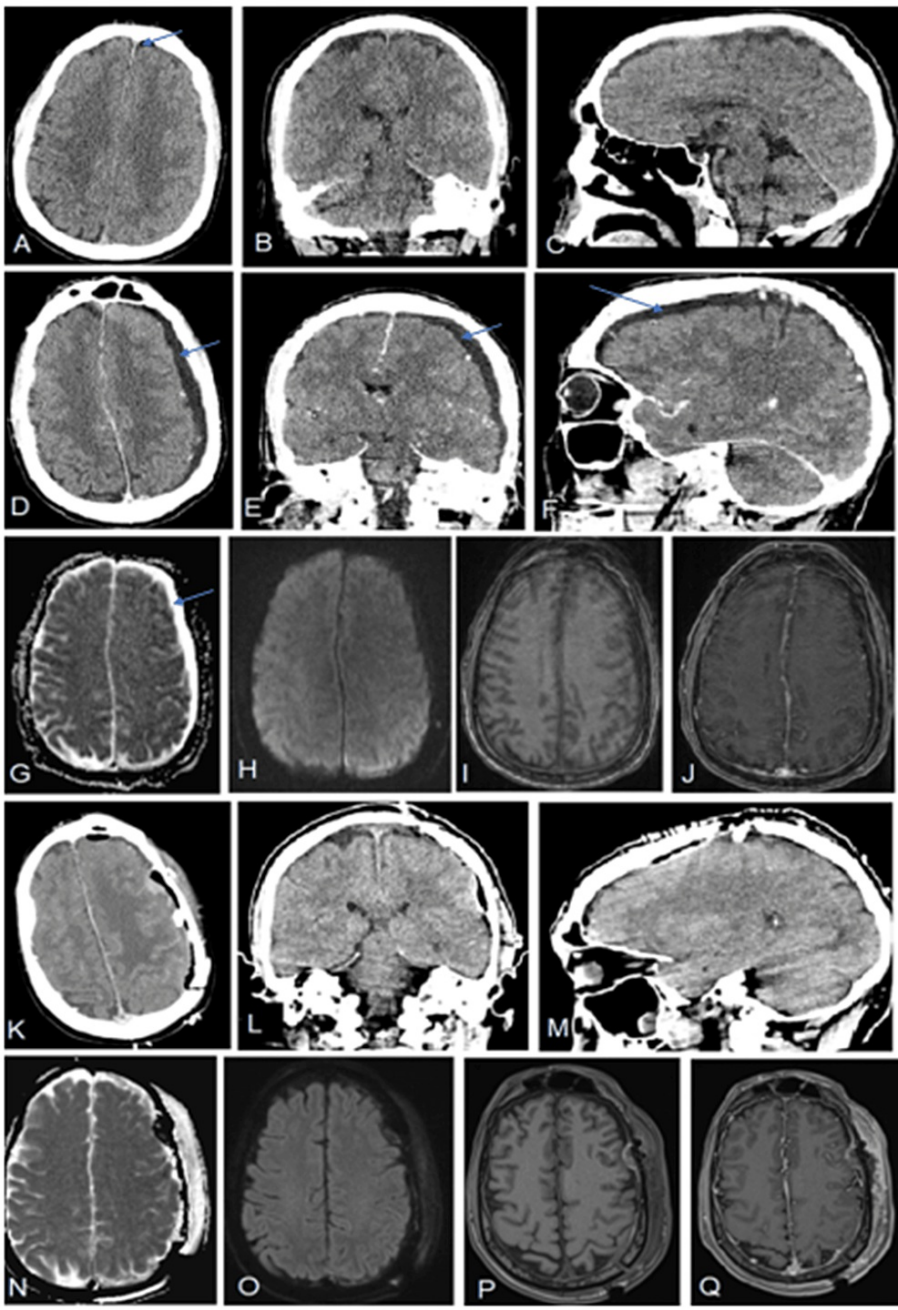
Radiographic images of a non-enhancing subdural empyema in a previously healthy 48-year-old male Figure 2: Each row, left to right reflects axial, coronal, and sagittal images. (A-C) Initial contrast-enhanced CT head demonstrating subtle left-sided hypodensities and trace pneumocephalus (blue arrow). (D-F) Repeat contrast-enhanced CT scan 72h later demonstrates interval development of a non-enhancing 5 mm left-sided hypodensity with significant mass effect (blue arrow). (G-J) Preoperative MRI; axial ADC (G) and DWI (H) sequences demonstrating a lack of diffusion restriction and T1-weighted axial images, without (I) and with gadolinium (J), demonstrating a lack of enhancement. (K-M) Postoperative CT head with contrast demonstrating interval left-sided craniotomy for subdural empyema evacuation. (N-Q) Postoperative MRI obtained five days later demonstrates a lack of diffusion restriction (N, O), overall improvement of mass effect, and no contrast enhancement in the surgical bed (P, Q). MRI: magnetic resonance imaging; DWI: diffusion-weighted imaging; ADC: apparent diffusion coefficient; CT: computed tomography
